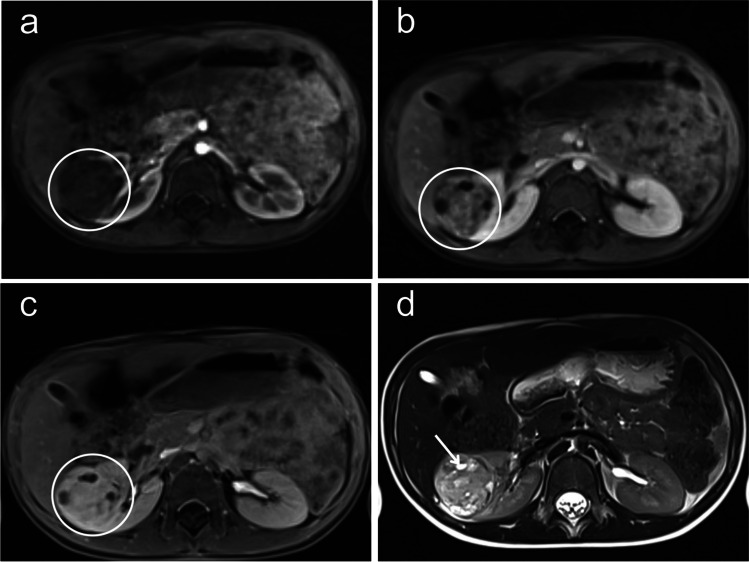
The contrast agent dynamics of the magnetic resonance images of the metanephric stromal tumor of a 5-year-old-boy shows relevant enhancement in the urographic phase only. a Dynamic axial T1-weighted volumetric interpolated breath-hold examination golden-angle radial sparse parallel (VIBE GRASP) magnetic resonance image with fat saturation shows delayed contrast agent uptake of the right-sided metanephric stromal tumor in the arterial phase compared to the renal parenchyma (*circle*). b The dynamic contrasted axial T1-weighted VIBE-GRASP magnetic resonance image shows the right kidney including the metanephric stromal tumor in the nephrographic phase with moderate enhancement. c The dynamic contrasted axial T1-weighted VIBE GRASP magnetic resonance image shows the right kidney including the metanephric stromal tumor in the urographic phase with enhancement comparable of the renal parenchyma. d Cystic (*arrow*) and solid parts are well demarcated in the axial T2-weighted half Fourier-acquired single shot turbo spin echo (HASTE) sequence of the magnetic resonance imaging
